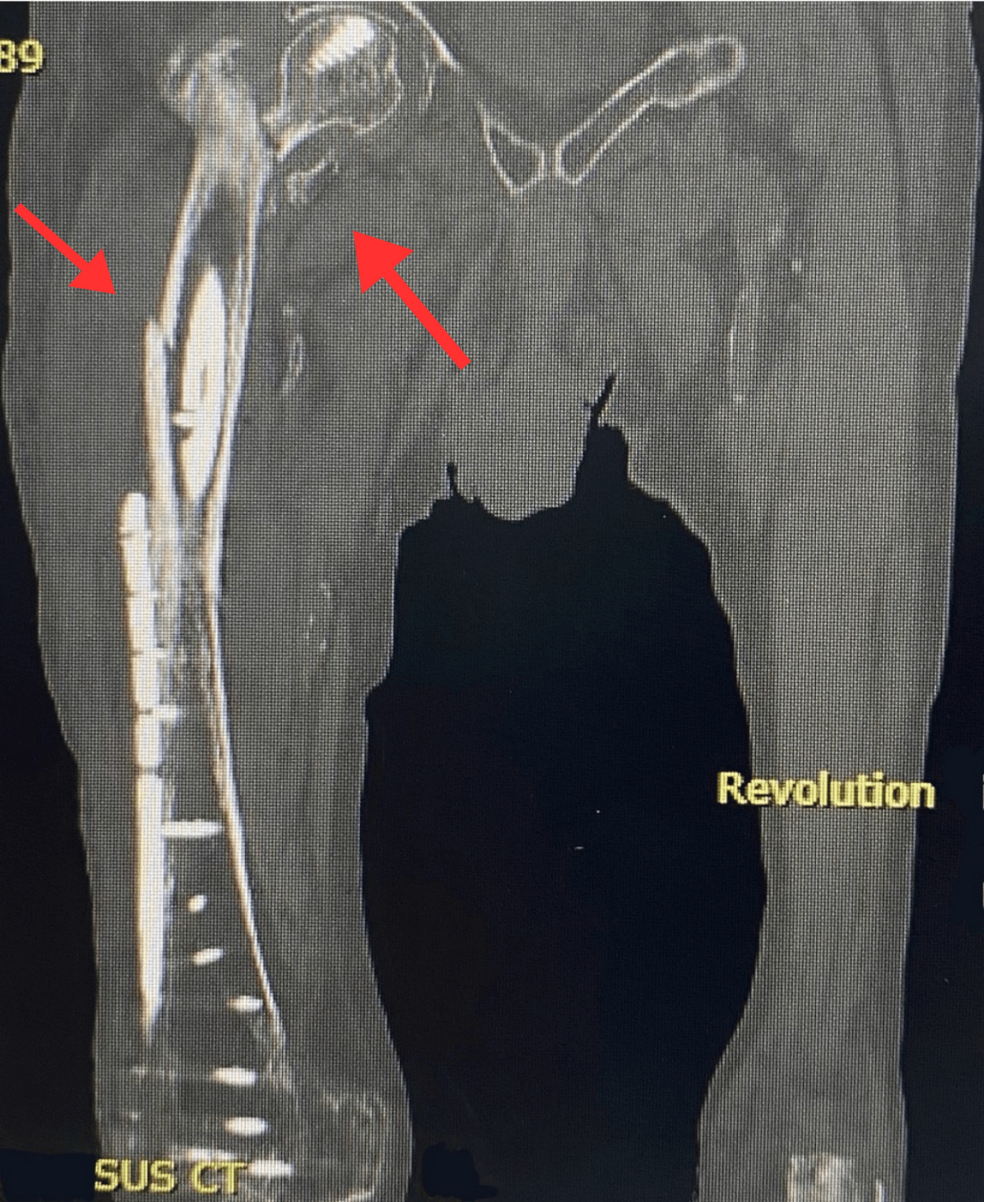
CT scan of the right femur, 17 June 2025, showing a proximal per-implant fracture near the TFNA hardware and evidence of varus malunion with shortening.
